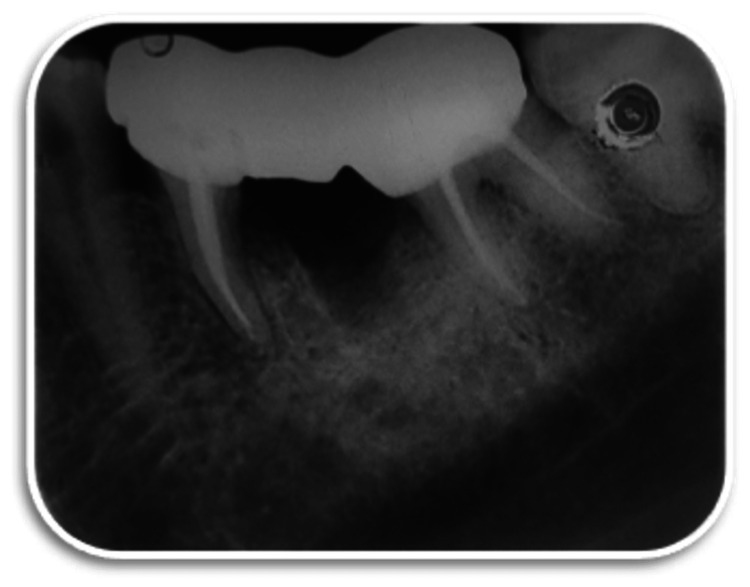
Three-month follow-up radiograph
